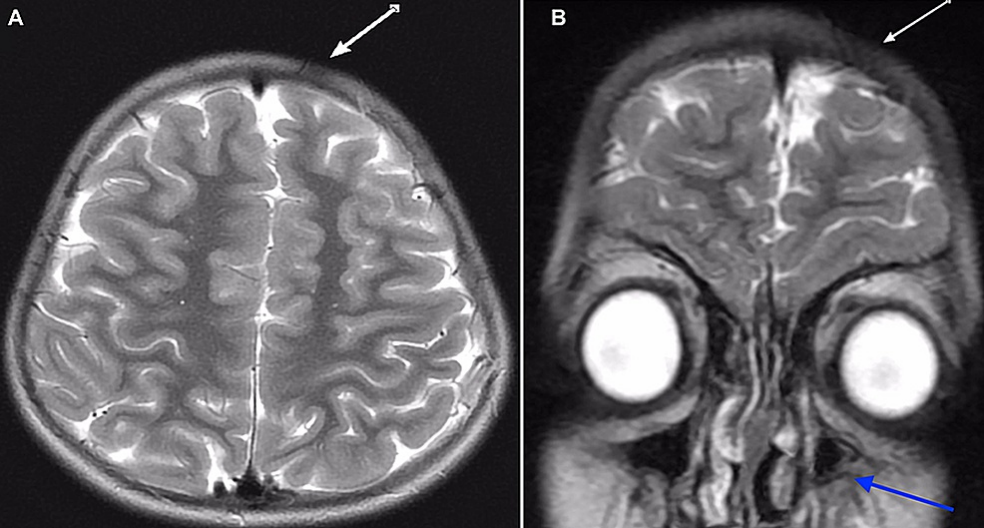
Sagittal and coronal T2-weighted magnetic resonance images demonstrate (A) a focal area of scalp thinning and subtle atrophy of the subcutaneous fat within the left frontal region without associated flattening or thinning of the underlying frontal bones and (B) no associated white matter abnormality. The left maxillary sinus is partially hypoplastic (blue arrow).
